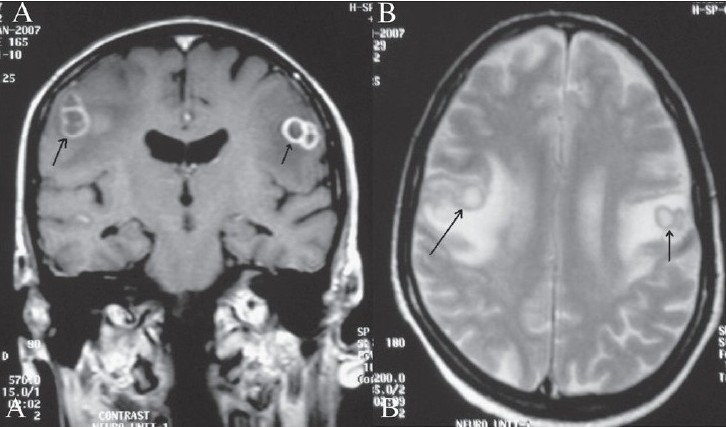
Contrast T1W coronal (A) and axial T2W MRI images of the brain show conglomerate ring-enhancing lesions in both the frontal subcortical regions (arrows in A), with central hyperintensity and peripheral low signal intensity rims (arrows in B)
